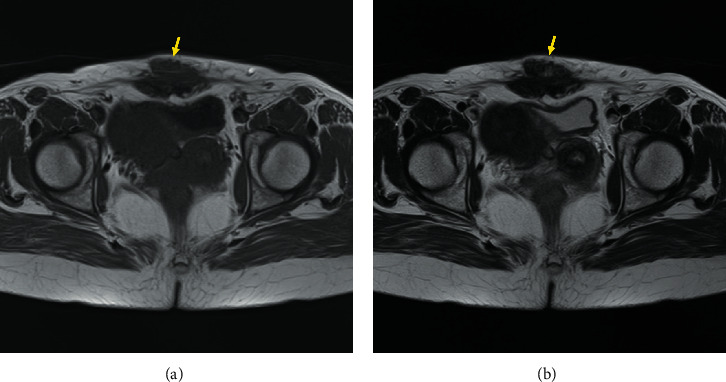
Preoperative MR images. (a) T1-weighted image. (b) T2-weighted image. A hypointense mass is observed within the rectus abdominis, as indicated by the yellow arrow. MR, magnetic resonance.
